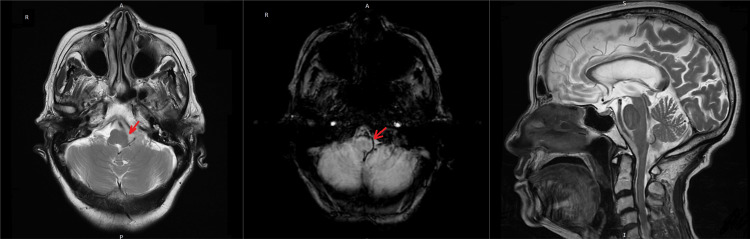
Brain MRI demonstrating subarachnoid hemorrhage Left: Axial T2-weighted MRI showing intermediate signal collection in the premedullary and left lateral cerebellomedullary cisterns (Red arrow). Middle: Axial susceptibility-weighted imaging (SWI) sequence demonstrating blooming artifacts consistent with subarachnoid hemorrhage (Red arrow). Right: Sagittal T2-weighted MRI showing the structural anatomy
